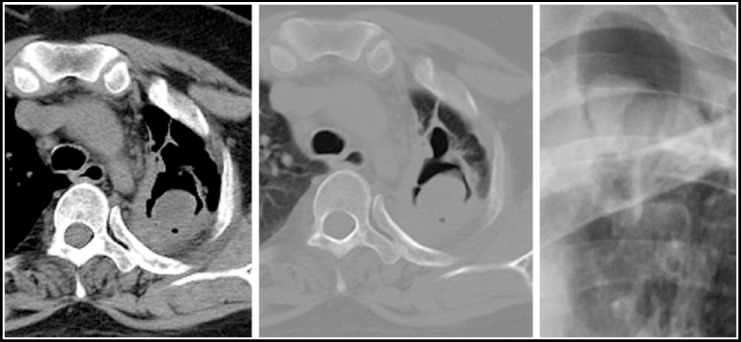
Chest X ray shows a cystic lesion with internal soft tissue opacity and air crescent sign. Corresponding CT chest sections in mediastinal and lung window settings shows a thin walled cavity with a fungal ball.
